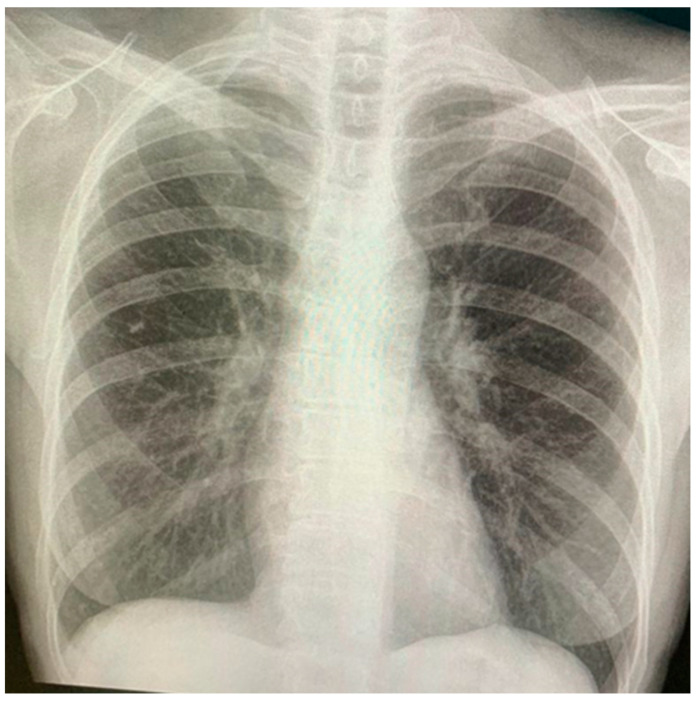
Posteroanterior chest X-ray seven months after the chemotherapy completion. Chest X-ray at posteroanterior projection. Well-positioned, well-inspired, and well-penetrated. Heart size is within normal limits. Lung fields are clear with no evidence of infiltrates. Hilar regions are unremarkable, and no pleural effusion was noted.
